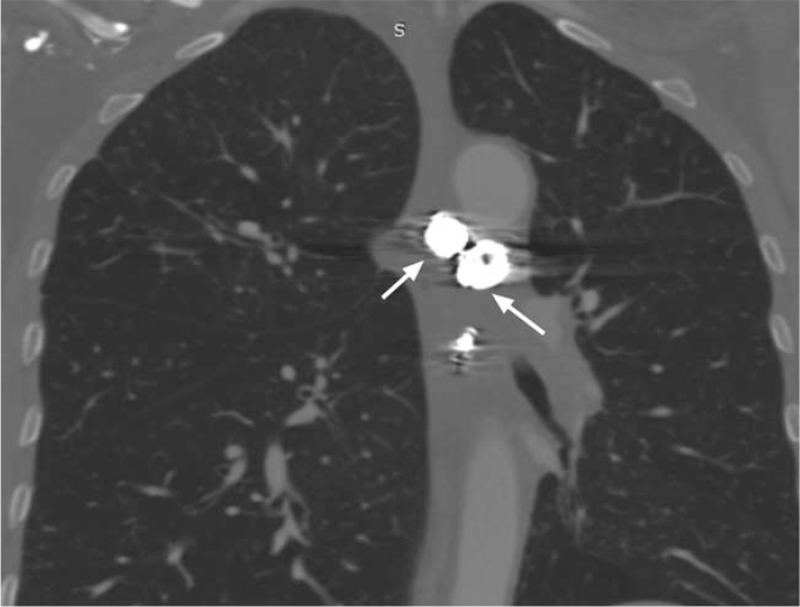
One-month follow-up CT angiography indicates that the coils were closely knit and in their proper position.
